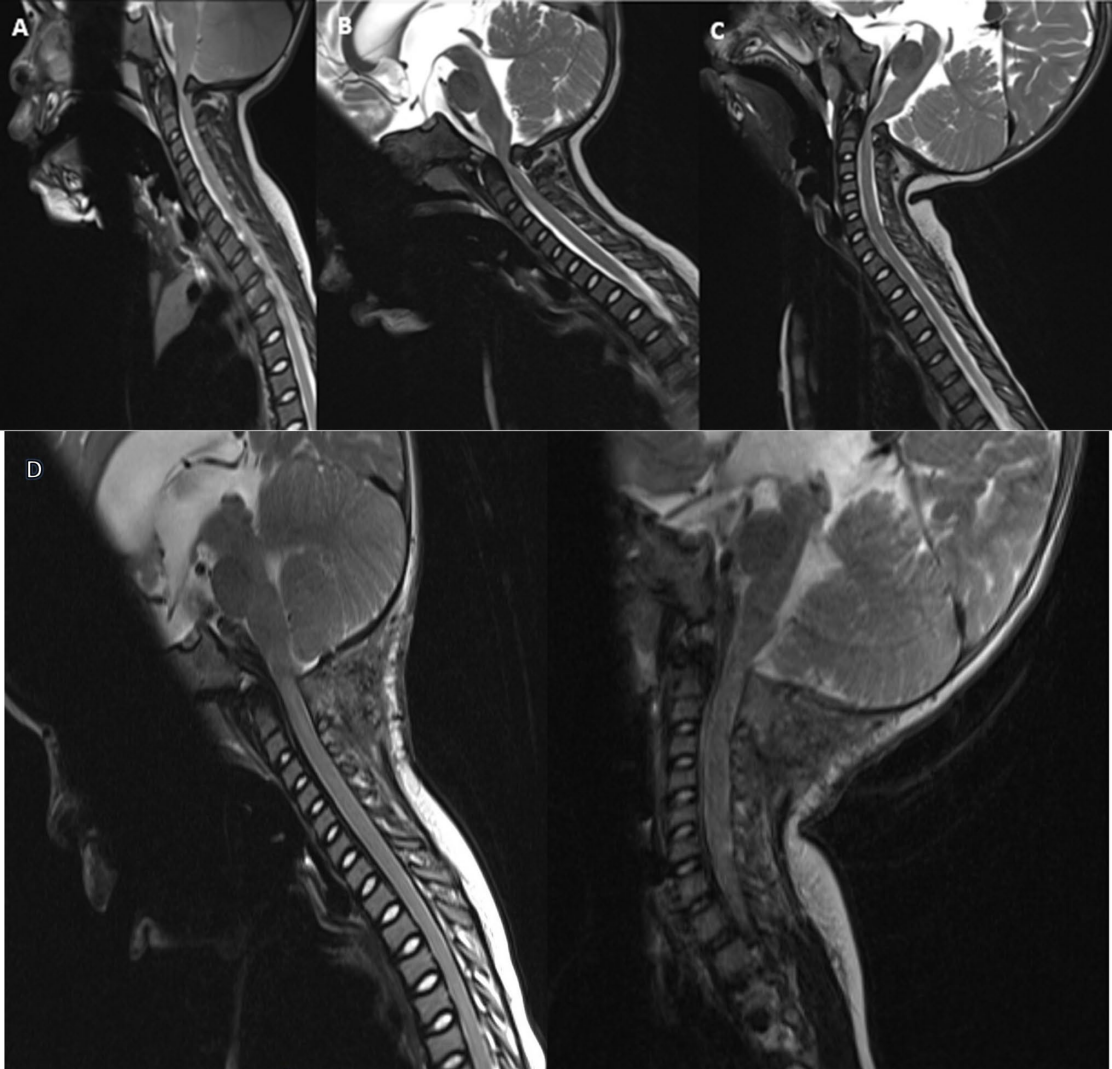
T2-weighted sagittal MRI of an 18-month-old female with achondroplasia, in neutral (A), flexion (B), and extension (C) positions demonstrating severe foramen magnum stenosis. D Post-surgical decompression results
